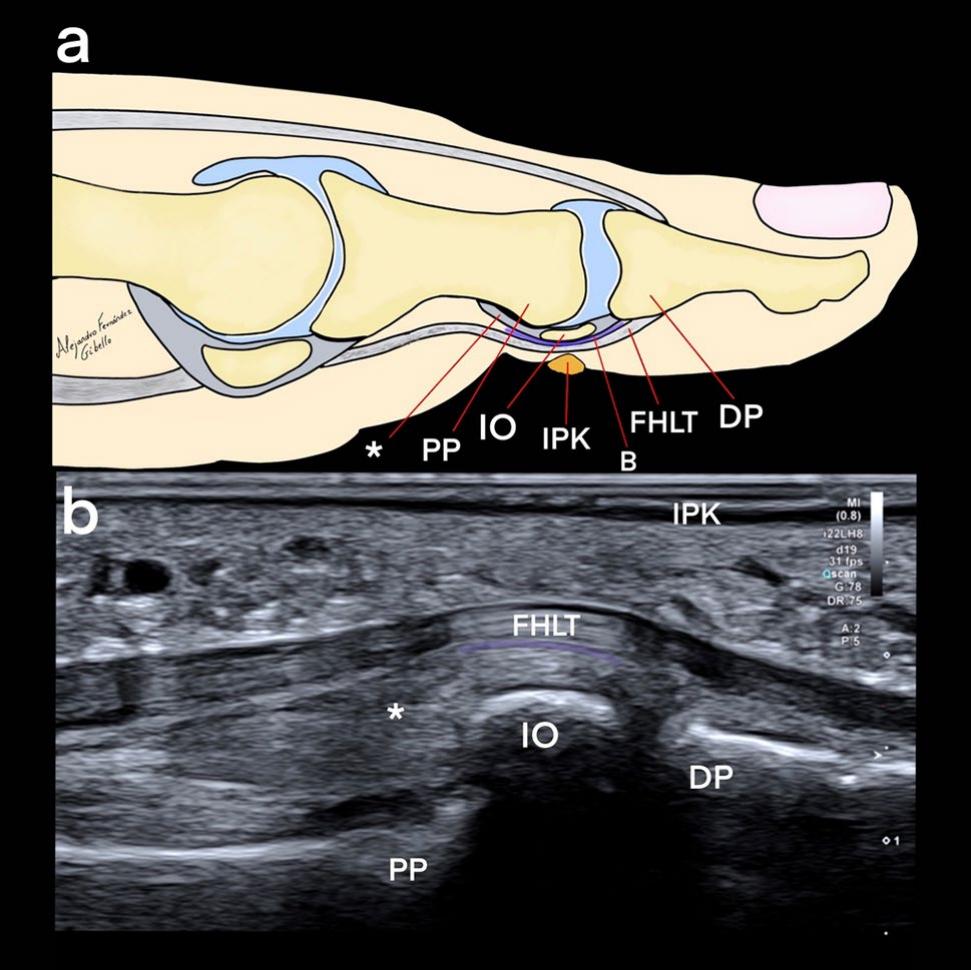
Comparison between a sketch (a) and an ultrasound sagittal view (b): *asterix plantar plate, *IO* interphalangeal ossicle, *PP* proximal phalangeal, *DP* distal phalanx, *FHLT* flexor hallucis longus tendon, *IPK* intractable plantar keratosis, *B* bursa.
